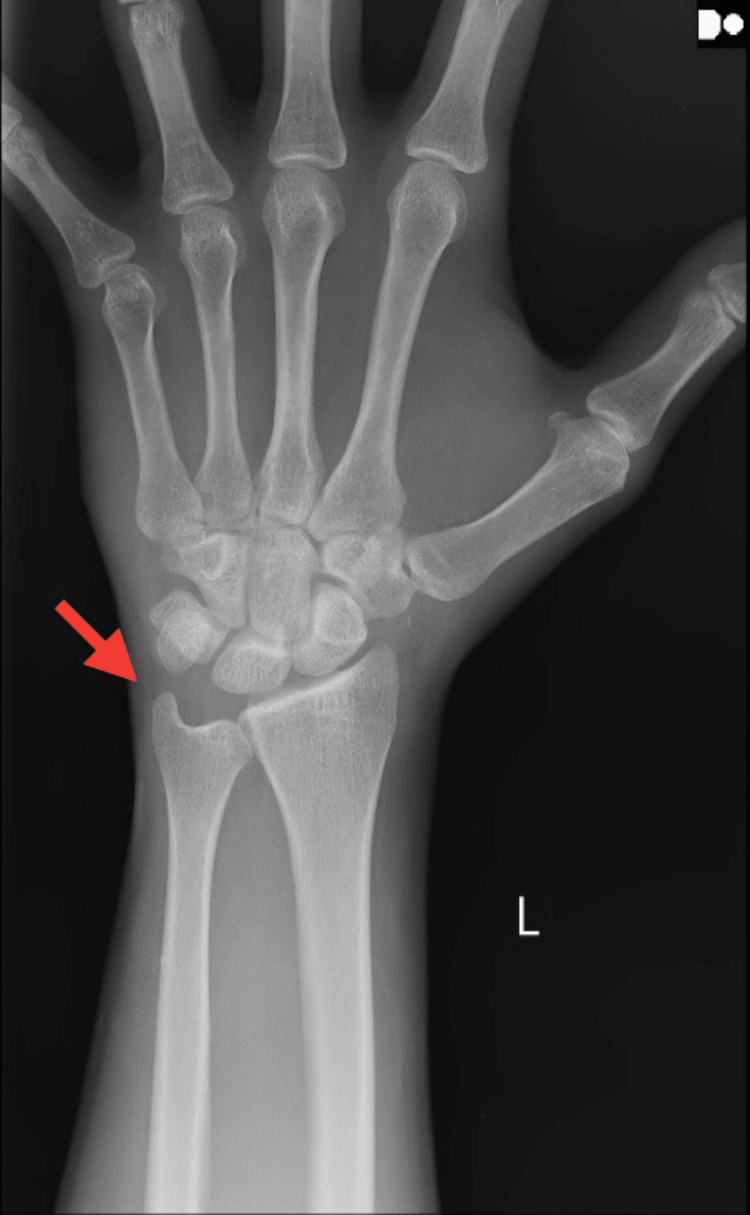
Radiograph of the left wrist in AP view showing negative ulnar variance AP, anteroposterior
